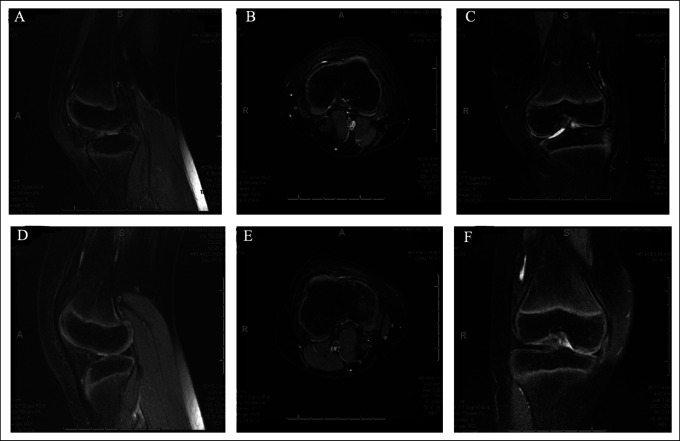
T2-weighted MRI scans of the right and left knee demonstrating bilateral absent patella. The rest of the ligamentous structures were intact. A, Left knee sagittal section, (B) left knee axial section, (C) left knee coronal section, (D) right knee sagittal section, (E) right knee axial section, and (F) right knee coronal section. MRI = magnetic resonance imaging.
